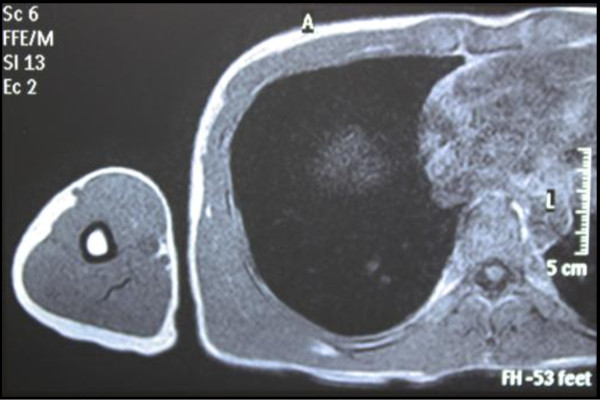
MRI image (T1-weighted). MRI imaging detected no lesion in the right thoracic wall.
